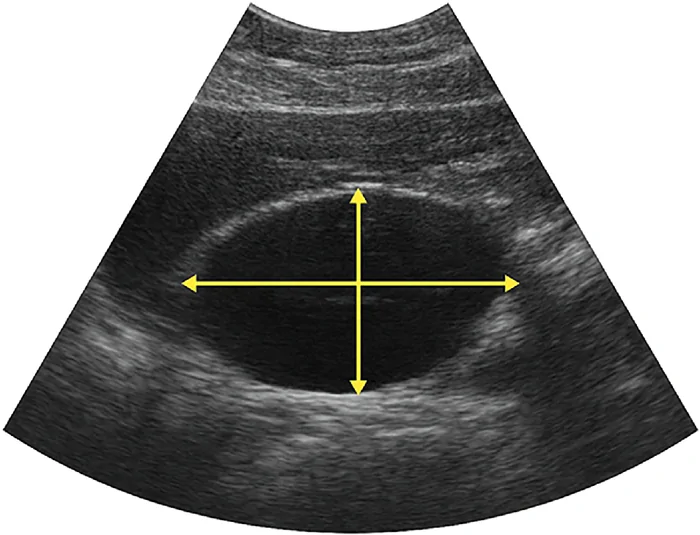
The antral cross-sectional area is depicted on a sagittal point-of-care ultrasound scan of the gastric antrum with the patient in the right lateral decubitus position. The yellow lines are ultrasound measurements of the gastric antrum to allow calculation of intragastric volumes. Gastric volume can be estimated from the right lateral decubitus antral cross-sectional area using the validated Perlas model.^11^ A simple bedside rule is if any antral diameter measurement exceeds 3 cm, the patient is likely to be high risk for pulmonary aspiration, although formal risk assessment should rely upon validated models and clinical context.
